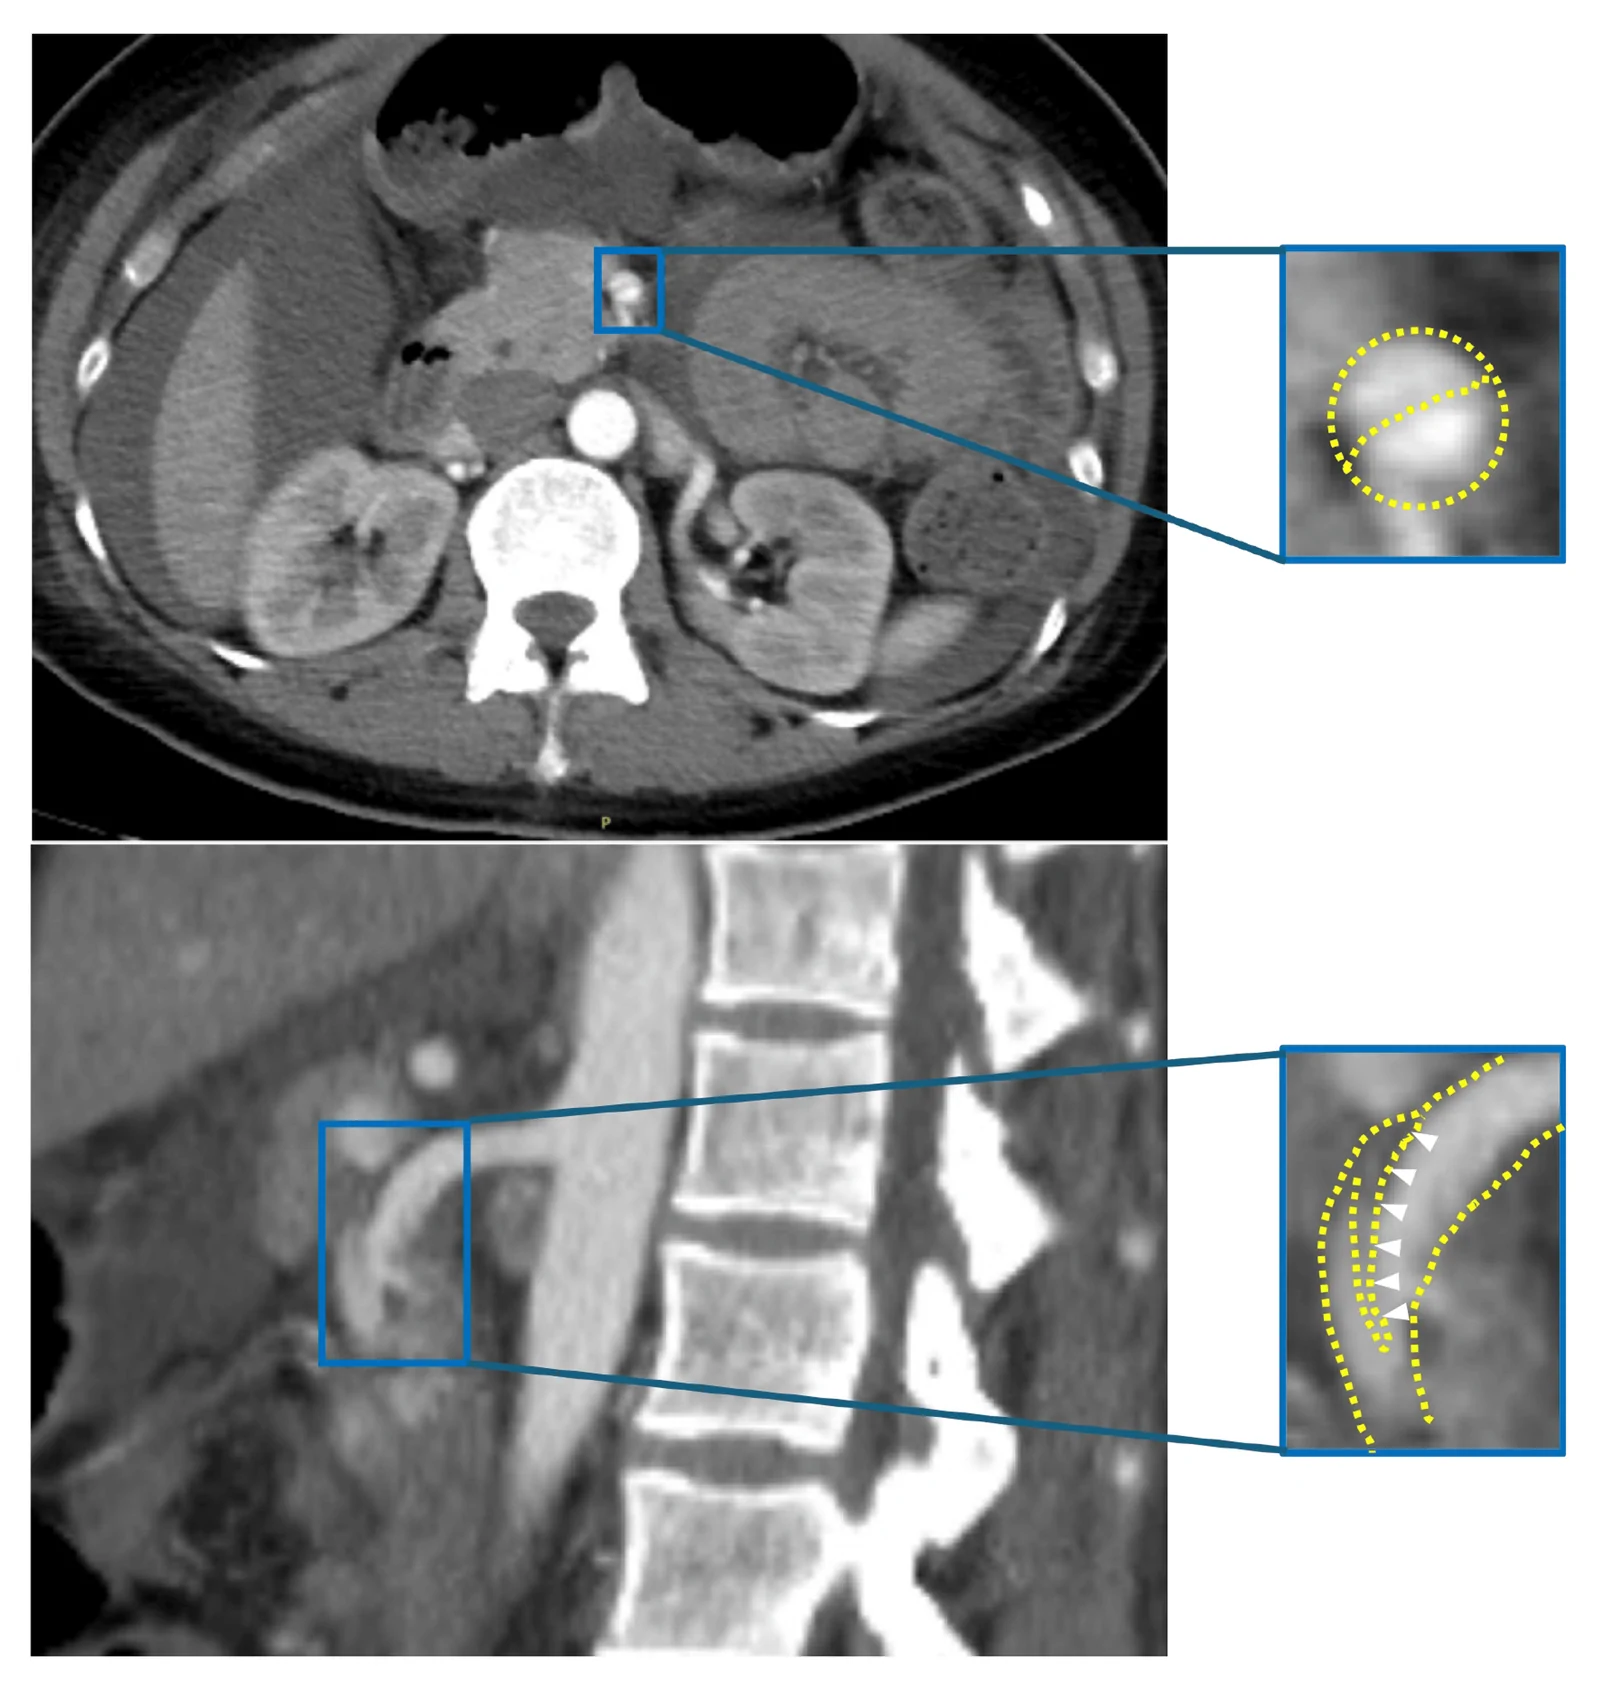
Contrast-enhanced computed tomography showing isolated superior mesenteric artery dissection after cesarean delivery. An intimal flap is visible within the superior mesenteric artery (arrowhead), consistent with arterial dissection. The distal branches remain patent, and there is no contrast extravasation or radiologic evidence of bowel ischemia.
